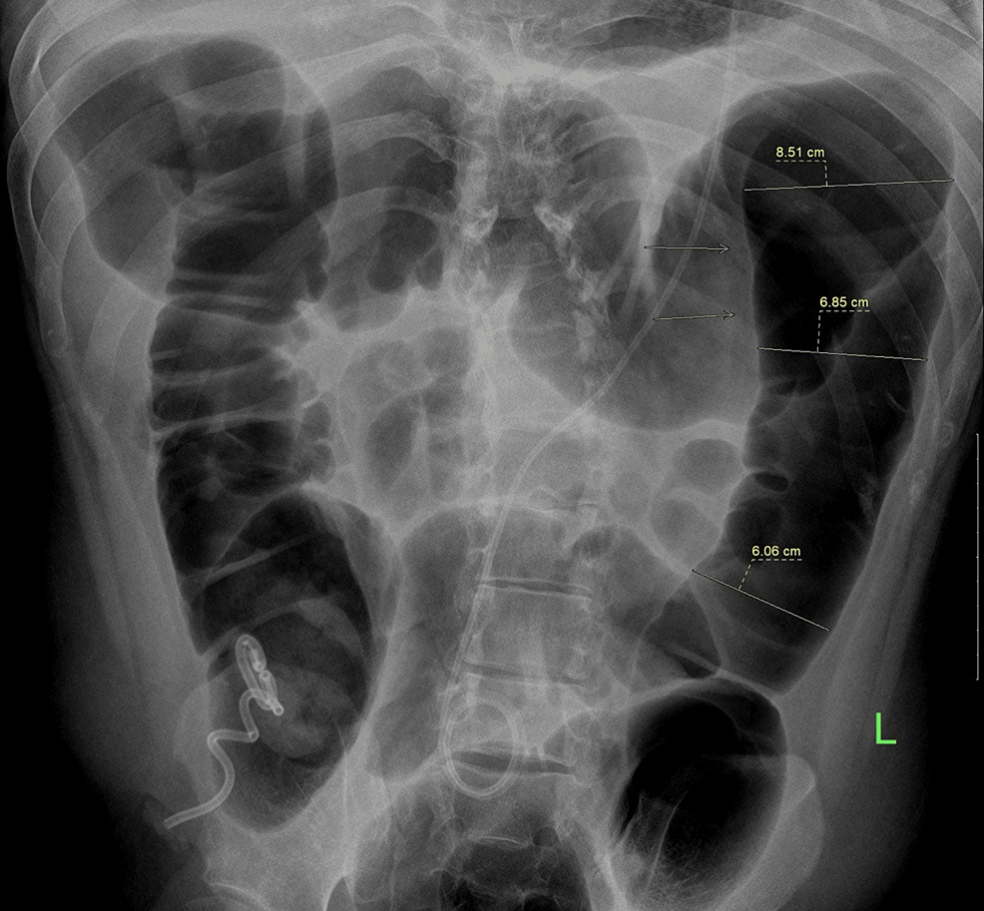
X-ray abdomen showing dilated large bowel loops
